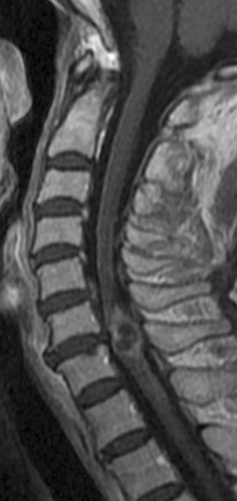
Sagittal T1-weighted magnetic resonance (MR) image with gadolinium revealing an intramedullary lesion with peripheral enhancement at the C6/7 level
